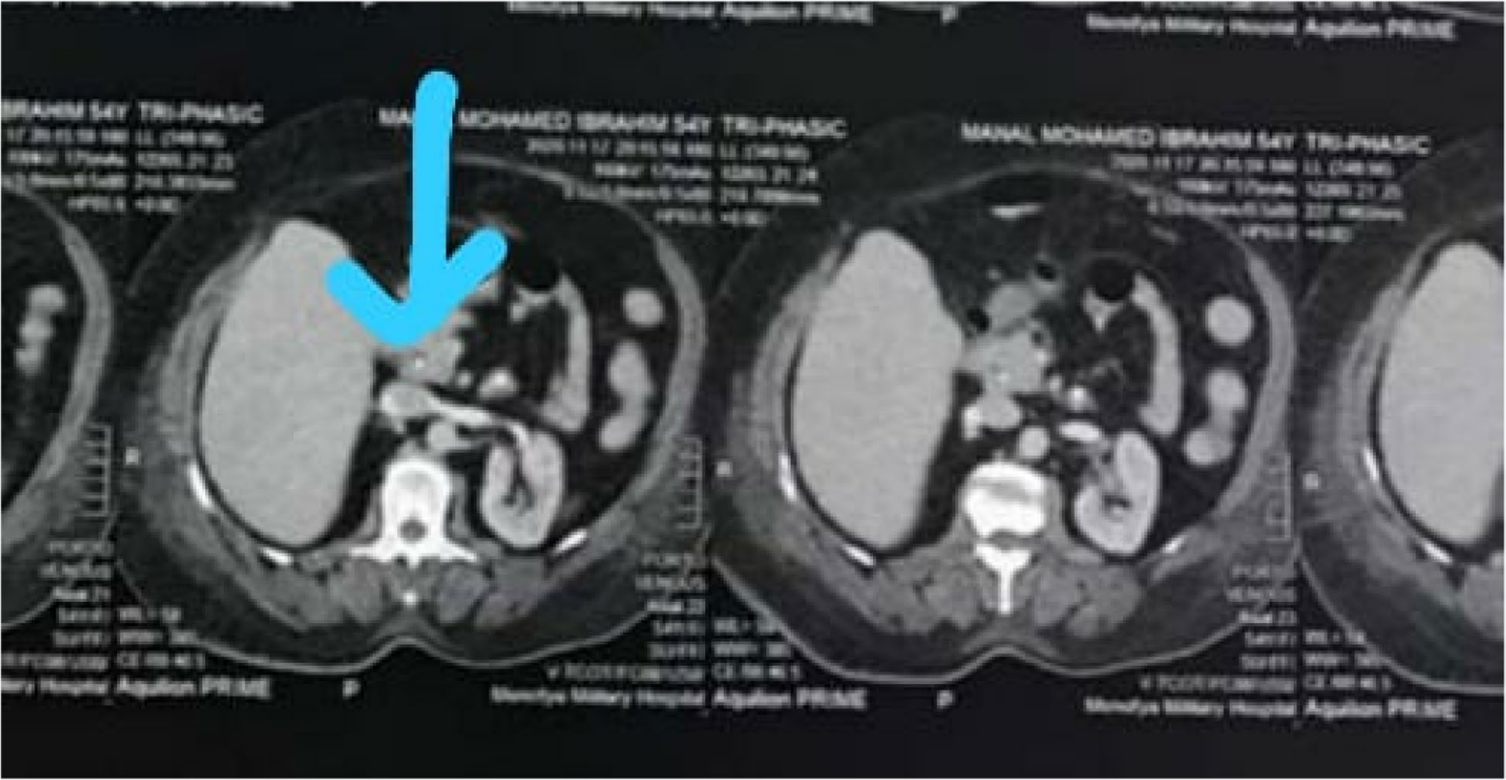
A faint thin stent hardly seen (atypical surgical catheter) on CT images
